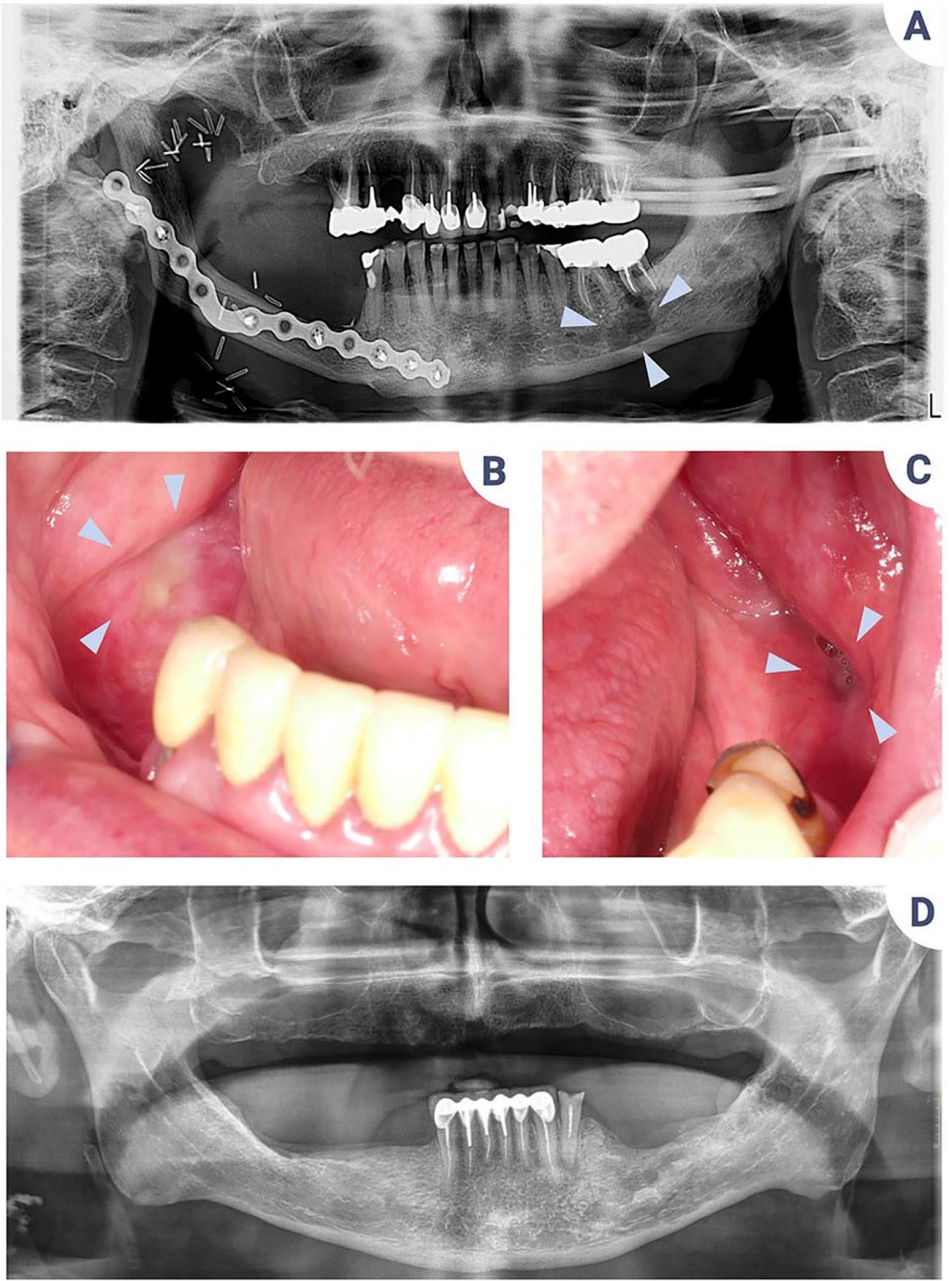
The intricacies of ORN diagnosis. A Panoramic X-ray of a patient with acinar cell carcinoma of the right submandibular gland treated in 2000 and recurrences in 2003, 2004, 2012, 2017, and 2020. Treatment consisted in multiple surgeries, radiotherapy, chemotherapy, and proton therapy. The clinical oral examination is complicated by the significant limitation of mouth opening. Tooth no. 37 clinically showed suppuration, suggestive of endodontic or periodontal infection, associated with a periapical radiolucency image (blue arrows). Given the patient’s history, she was referred to the oncology team for an opinion. The diagnosis of ORN was confirmed. B–D Clinical views and panoramic X-ray of a patient with a history of squamous cell carcinoma of the right tonsil treated by radiochemotherapy in 2007. He presented with two foci of ORN in the posterior mandibular sectors, suggesting traumatic mucosal ulceration due to his removable dental prosthesis. Early diagnosis and drug therapy resulted in stabilization of the situation
